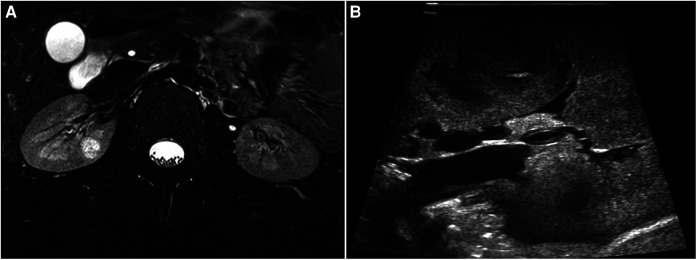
(A) Pre-operative MRI of renal lesion within the right kidney parenchyma. (B) Lesion localization with intraoperative ultrasound.
